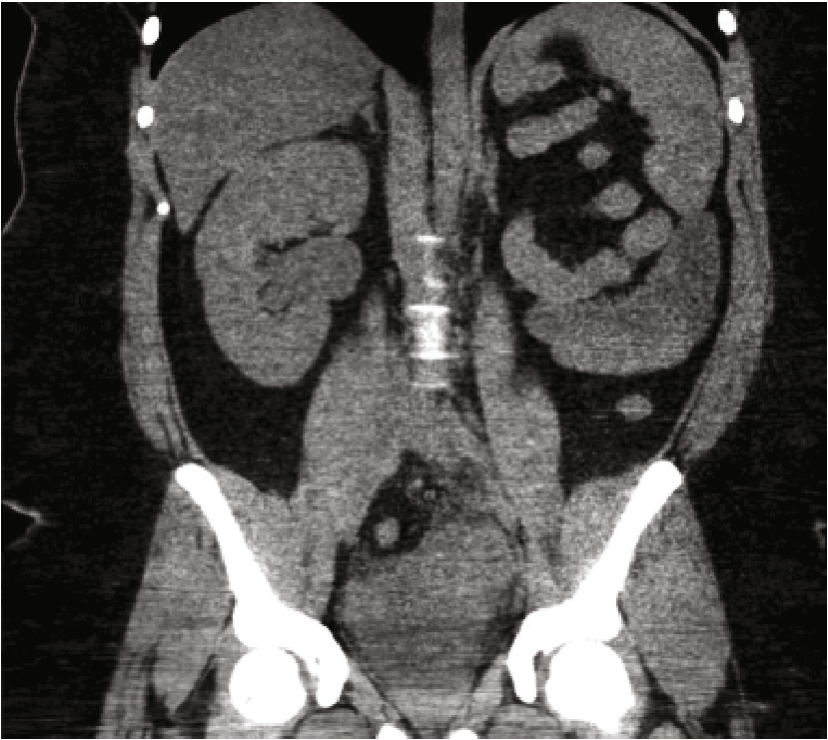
Coronal CT of the abdomen and pelvis without contrast demonstrates a normal appearing right kidney. The left kidney is not visualized which is consistent with left renal agenesis.
